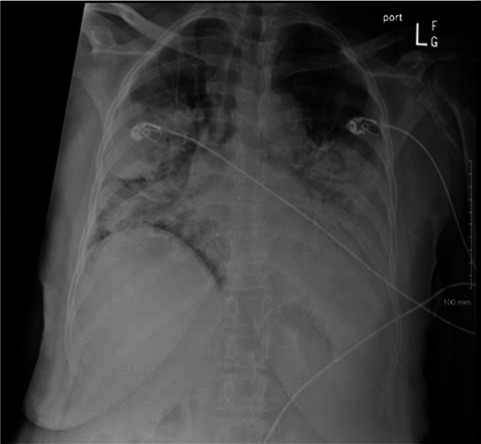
Chest X-ray: bilateral consolidations.
